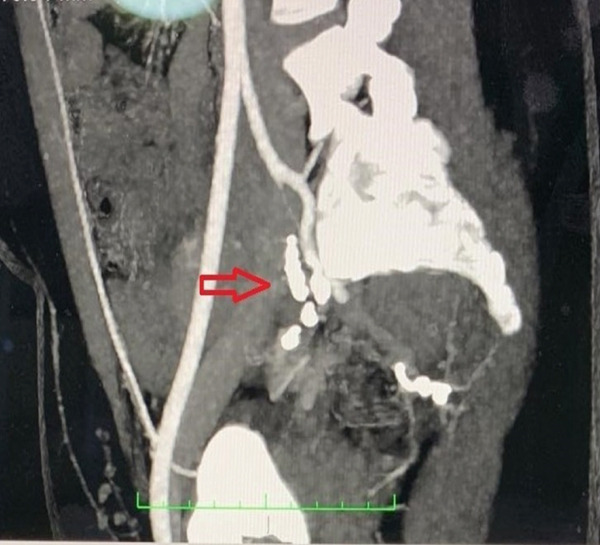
Follow‐up contrast‐enhanced computed tomography (CT) imaging performed at 1‐month postselective uterine artery embolization demonstrates stable postprocedural findings. Sagittal and coronal reformatted views reveal the presence of embolization coils at the previously treated site of the uterine artery pseudoaneurysm (denoted by red arrows). There is no evidence of active contrast extravasation, residual perfusion, or recurrence of the pseudoaneurysm.
